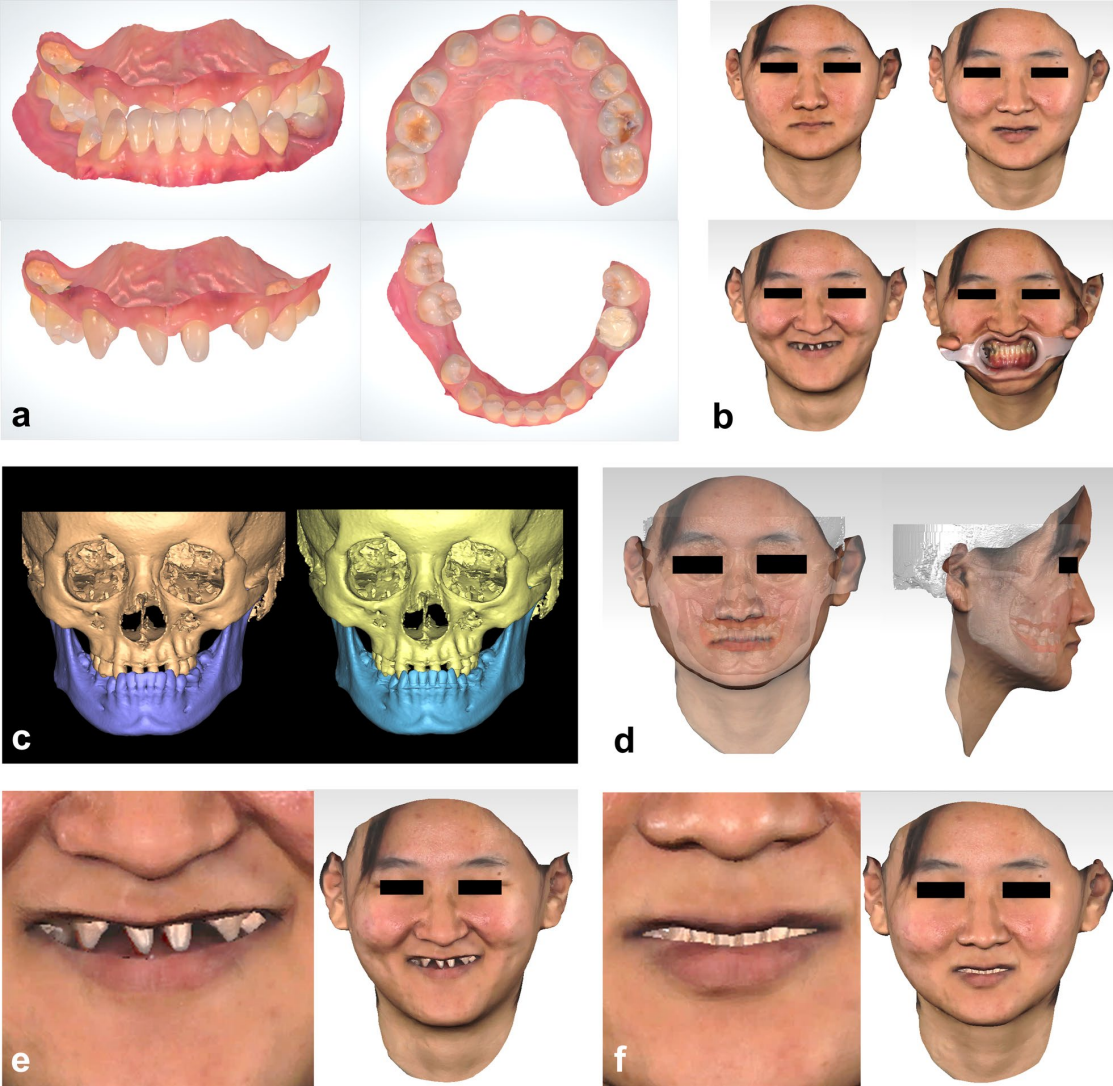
Initial 3D data acquisition and patient digitalization. a 3D dentitions; b 3D facial photographs in rest position, wide smile, closed lips and maximum intercuspal position; c 3D information of oral-maxillofacial bones from CBCT (left) and the teeth are replaced with 3D dentitions (right); d–f 3D virtual patient in closed lips (d), wide smile (e) and rest position (f)
